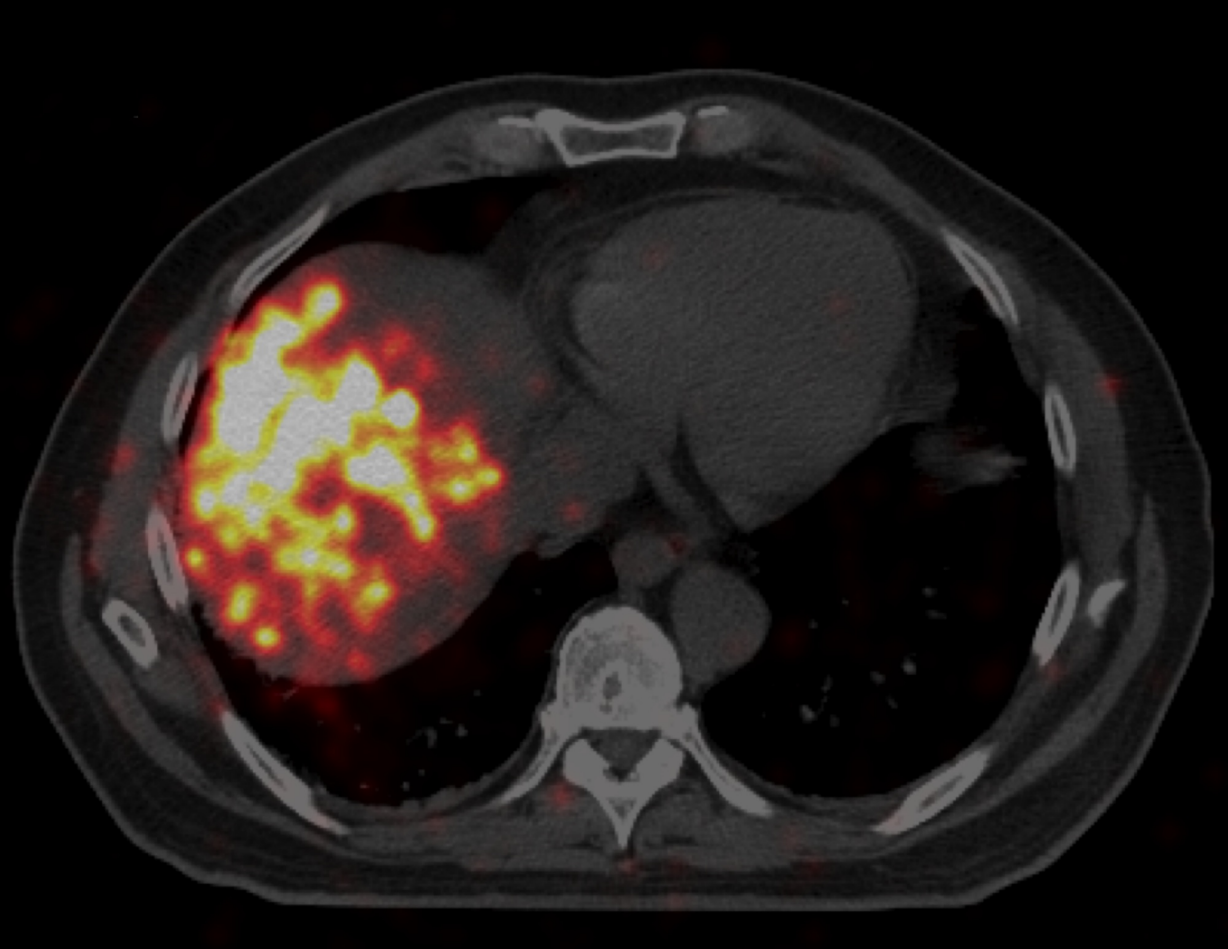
July 2017 PET/CT (scan of Y-90 activity) two hours post Y-90 PET: positron emission tomography, CT: computed tomography
